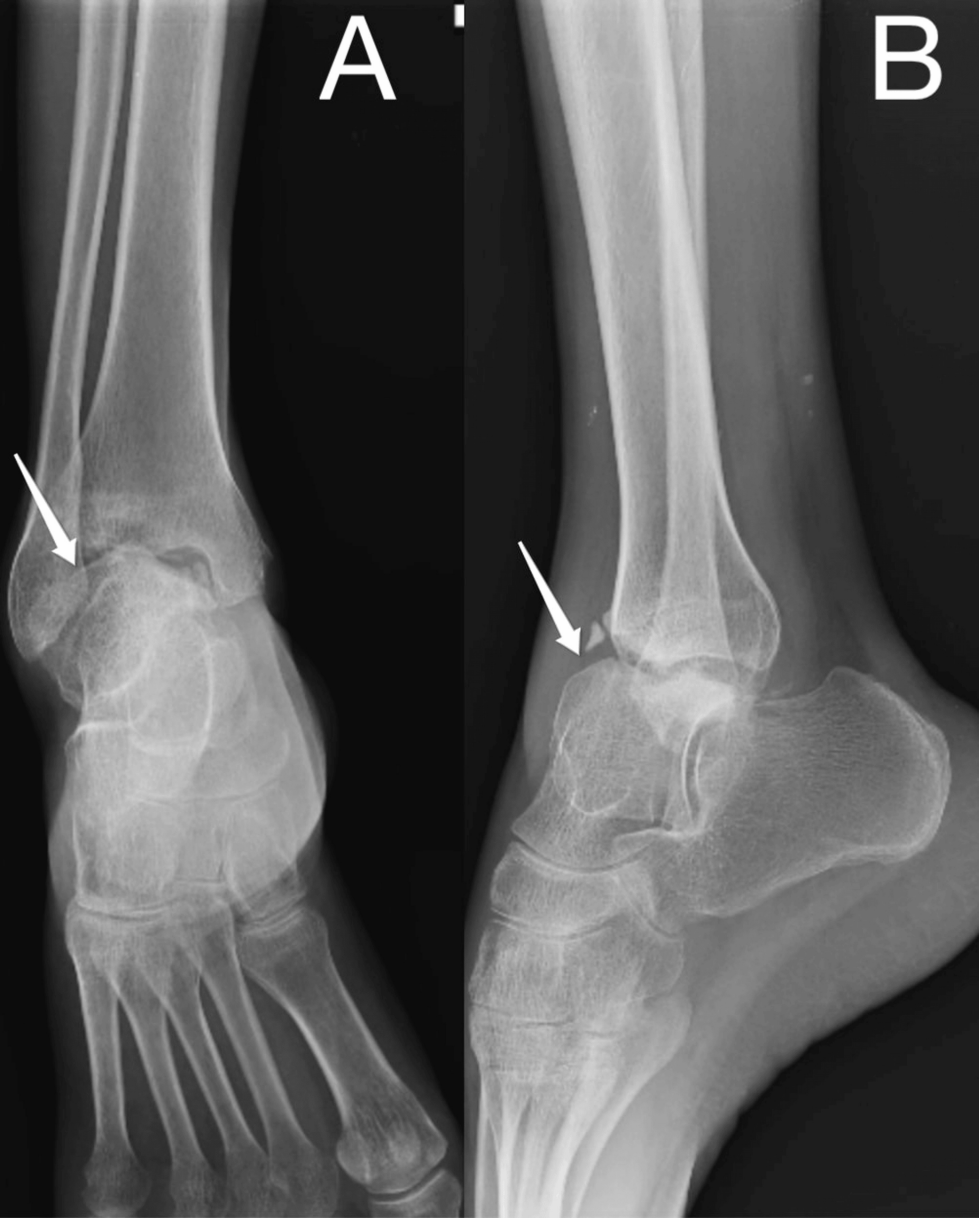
Preoperative radiograph of the right ankle (A) anteroposterior view and (B) lateral view. The white arrows show the subluxation of the talus
